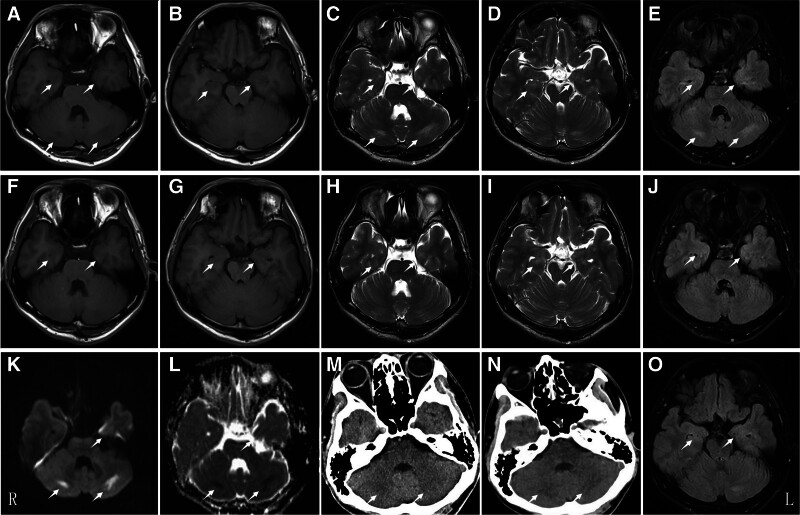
Cranial MRI (A–J,O) 29 hours after onset revealed bilateral cerebellum and hippocampus with low signal in T1 and ADC and high signal in T2, T2-FLAIR and DWI (white arrows). Follow-up brain MRI (F–E,K,L) showed slightly low signal in T1, high signal in T2, and T2-FLAIR in bilateral hippocampus 4 mo later. Cranial CT 19 h after onset (N) showed more visible and lower signal than that 1.5 h after onset (M). ADC = apparent diffusion coefficient, DWI = diffusion-weighted inversion recovery, MRI = magnetic resonance imaging.
